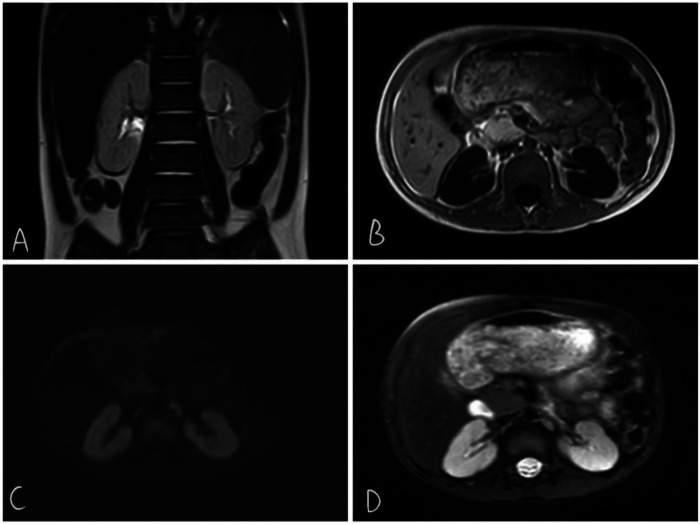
T2wi coronal view shows somewhat poorly defined corticomedullary boundaries in both kidneys (A) T1WI shows long TIWI signals in both kidneys (B) DWI shows no restriction of diffusion (C) T2WI signal shows slightly increased cortical signal in T2WI (D)
